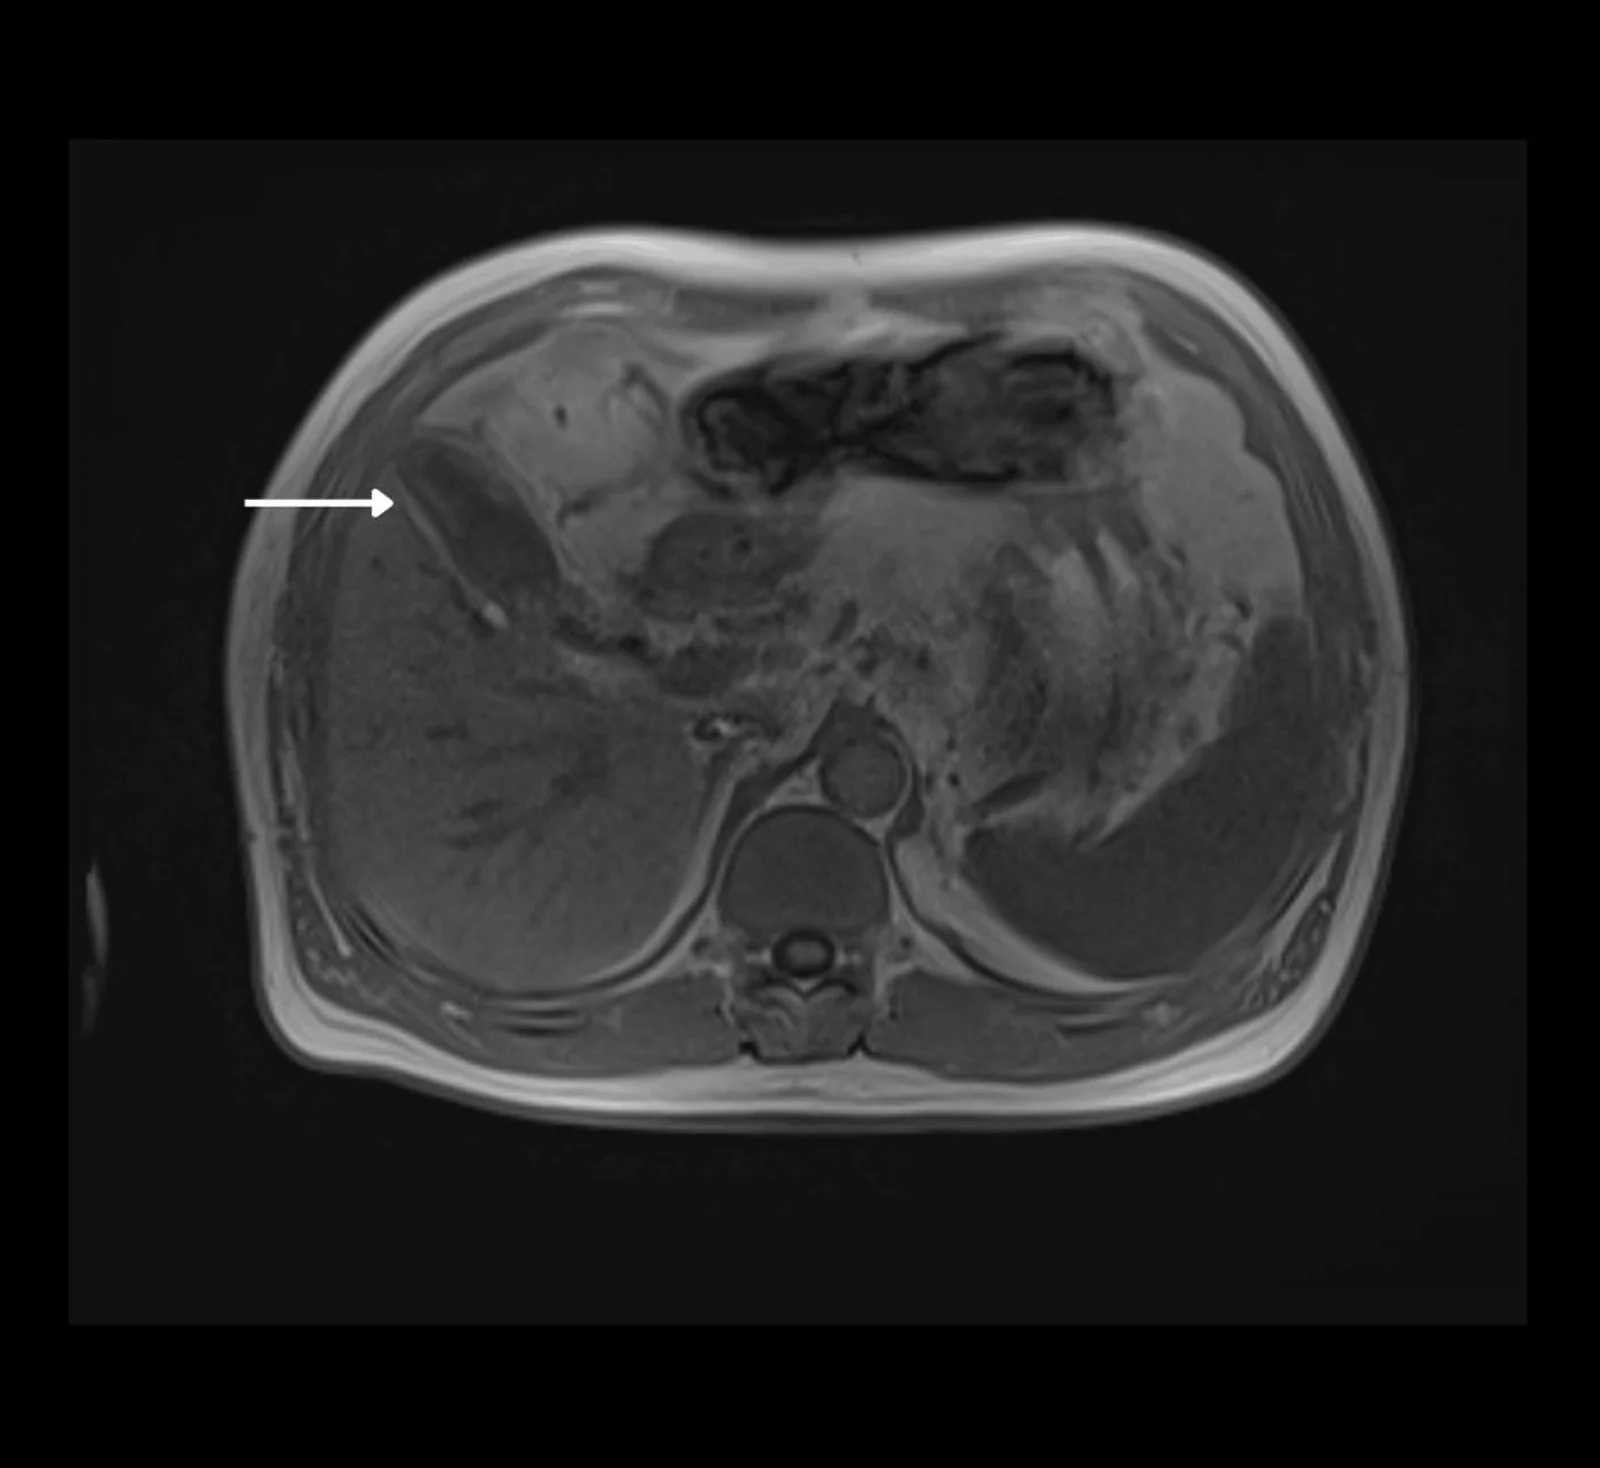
MRCP T1-weighted axial image showing focal asymmetric hypointense wall thickening involving the fundus and distal body of the gallbladder, measuring up to 6 mm The arrowhead points toward the focal wall thickening at the fundus.
